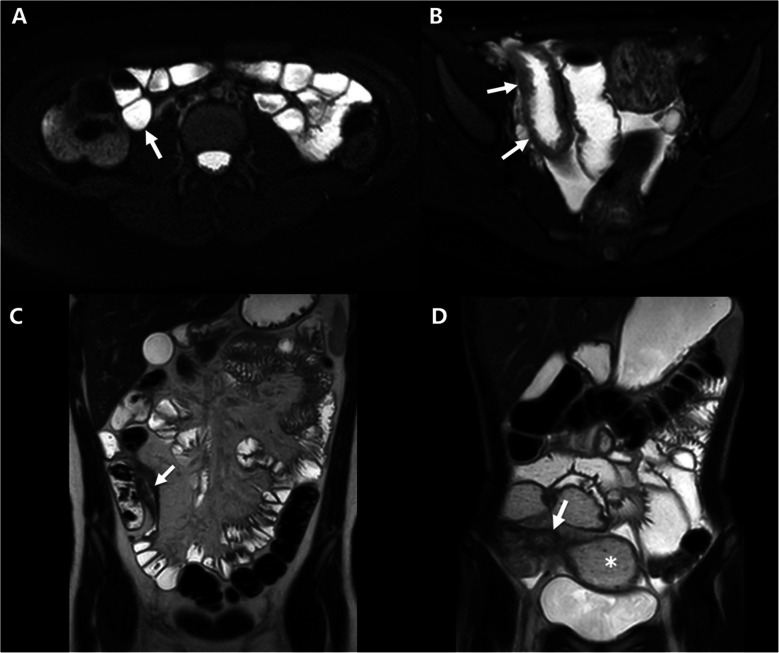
Examples of MRI images of the AGA-SAR classification system. a A 13-year-old with Crohn’s disease. Axial T2-weighted single-shot fast spin-echo image of the terminal ileum (*arrow*) appears normal without wall thickening or luminal narrowing. b A 16-year-old with Crohn’s disease. Axial T2-weighted single-shot fast spin-echo image shows terminal ileal wall thickening (*arrows*), but no luminal narrowing. There is also intramural edema and mucosal ulcers. c A 16-year-old with Crohn’s disease. Coronal T2-weighted single-shot fast spin-echo image shows terminal ileal wall thickening and luminal narrowing (*arrow*), but no dilation of the upstream bowel. d A 14-year-old with Crohn’s disease. Coronal T2-weighted single-shot fast spin-echo image shows terminal ileal wall thickening and luminal narrowing (*arrow*) as well as upstream dilation of the more proximal ileum and the small bowel feces sign (*asterisk*)
